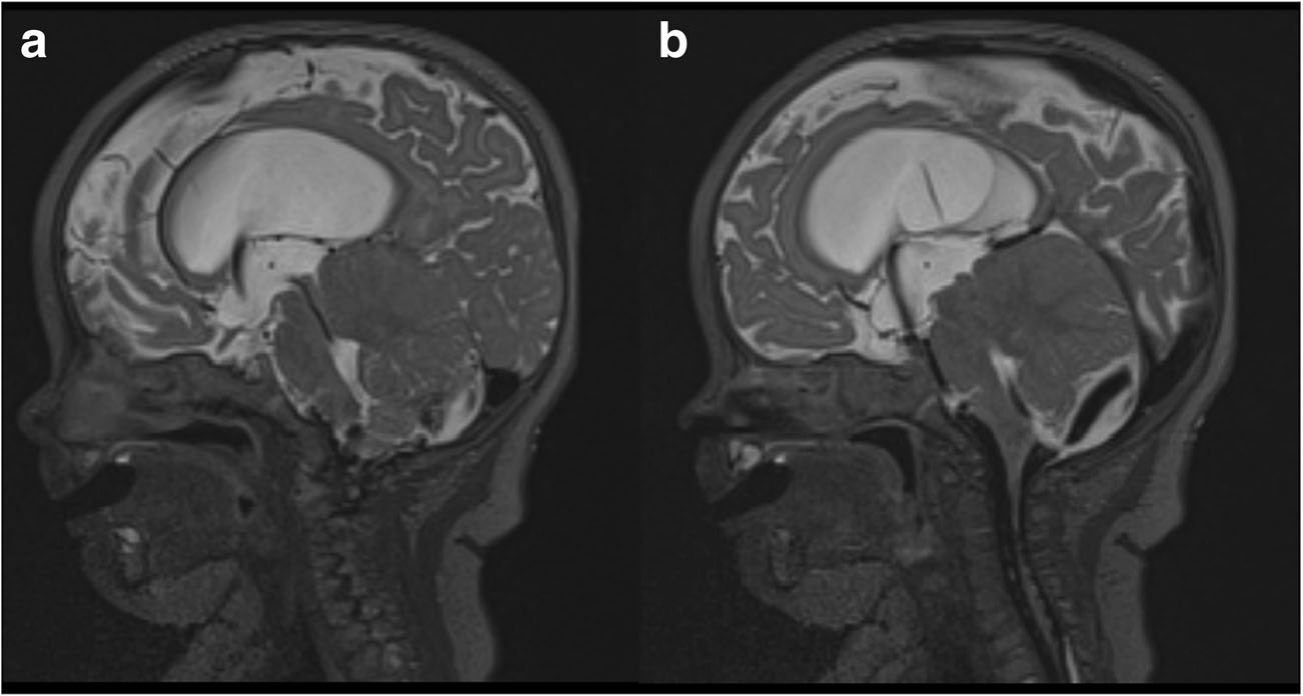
Postoperative MRI scan demonstrating reduction in size of posterior fossa AC after fenestration; sagittal view with flow through the aqueduct a; sagittal view with flow through ventriculostomy and outlets of the 4^th^ ventricle b
